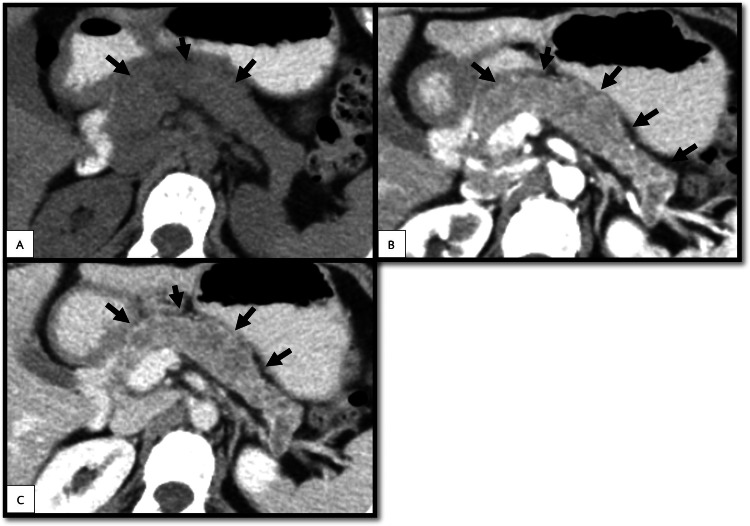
Abdominal computed tomography (CT) scan (arrow, the tumor) Unenhanced CT scan (A) revealed an isoattenuating to slightly low-attenuating tumor. Contrast-enhanced CT (B, arterial phase; C, portovenous phase) revealed the hypovascular tumor inside the dilated main pancreatic duct.
